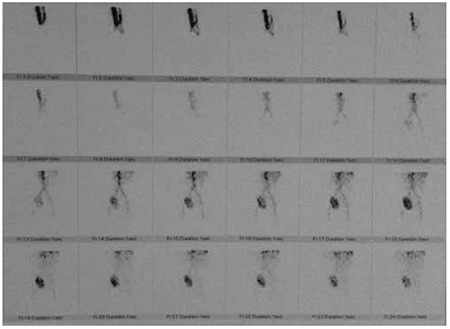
Dynamic renal scintigraphy, anterior view after bolus injection of 7 mCi 99mTechnetium-diethylenetriaminepentaacetic acid through an 18-gauge venous cannula in the right antecubital vein. In the angiographic phase, sequential 1-second images revealed an unusual vascular filling pattern along with patent iliac arteries and the transplanted kidney
